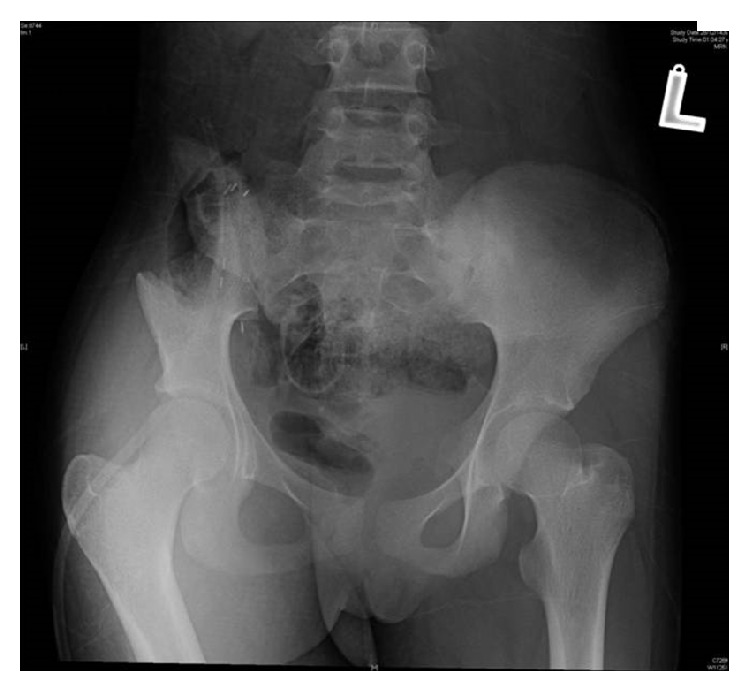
X-ray image of the patient showing right internal hemipelvectomy.
